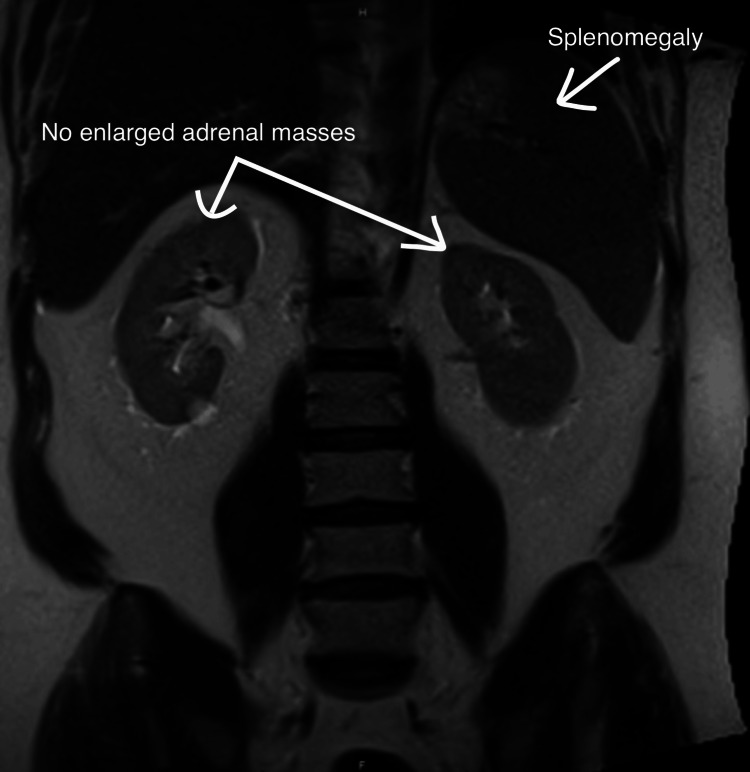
T2-weighted (T2W) MRI abdomen and pelvis post treatment No evidence of lymphoma relapse.
